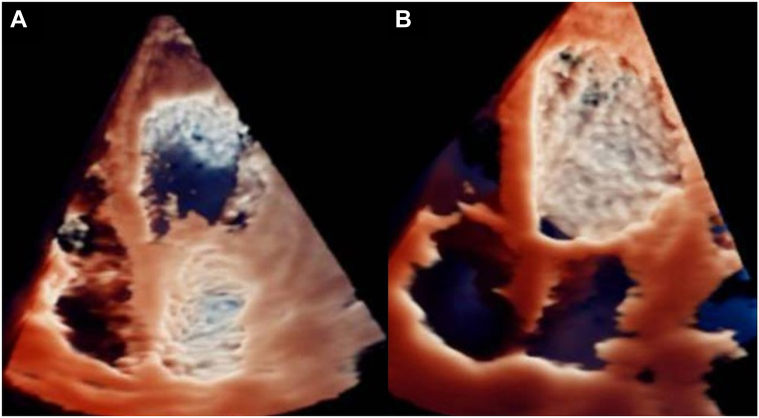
Baseline and Follow-Up Transthoracic Echocardiography (TrueVue) (A) Apical 4-chamber view showing obliteration of the left ventricular apex by intracavitary echogenic material, with reduced ventricular volumes. (B) Marked reduction of the intracavitary echogenic material after several months of imatinib therapy.
